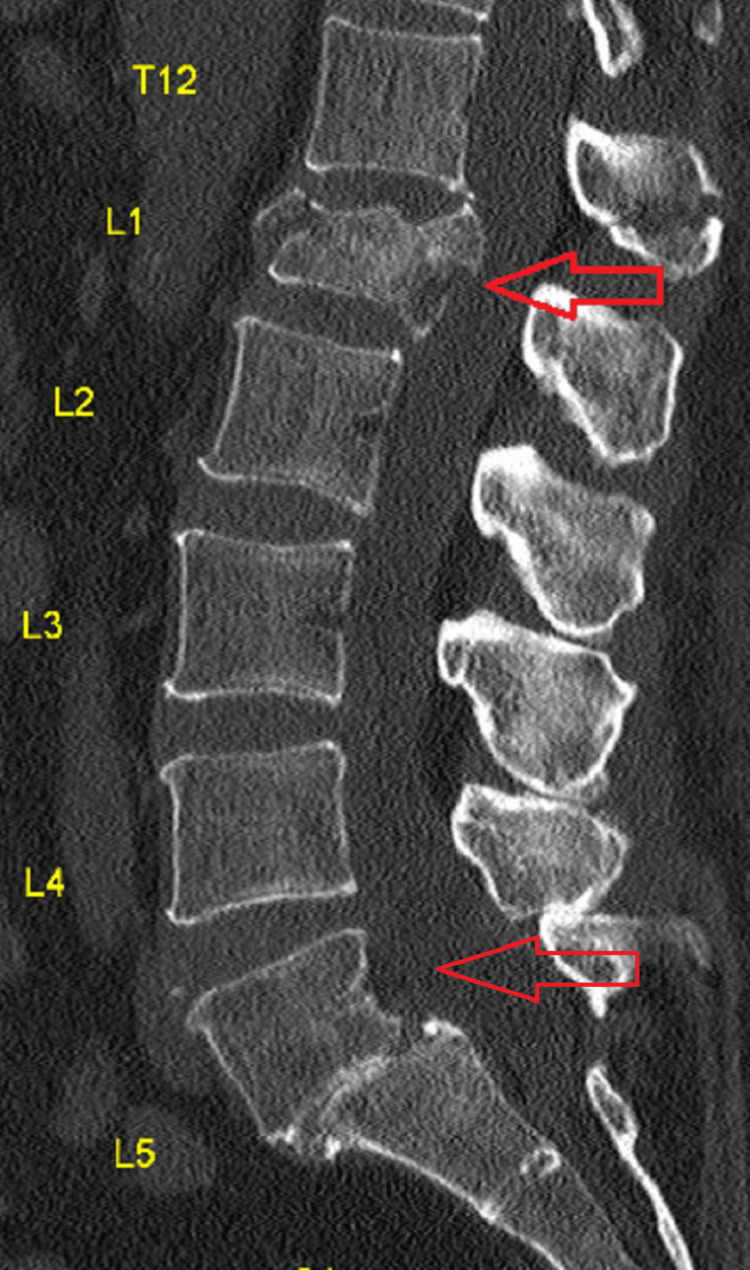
CT scan of the lumbosacral region from the sagittal plane, revealing evidence of a compression fracture of L1 alongside decreased intervertebral disc space and spondylolisthesis in the lumbosacral junction (L5/S1), with various degenerative changes of the lumbar vertebrae
